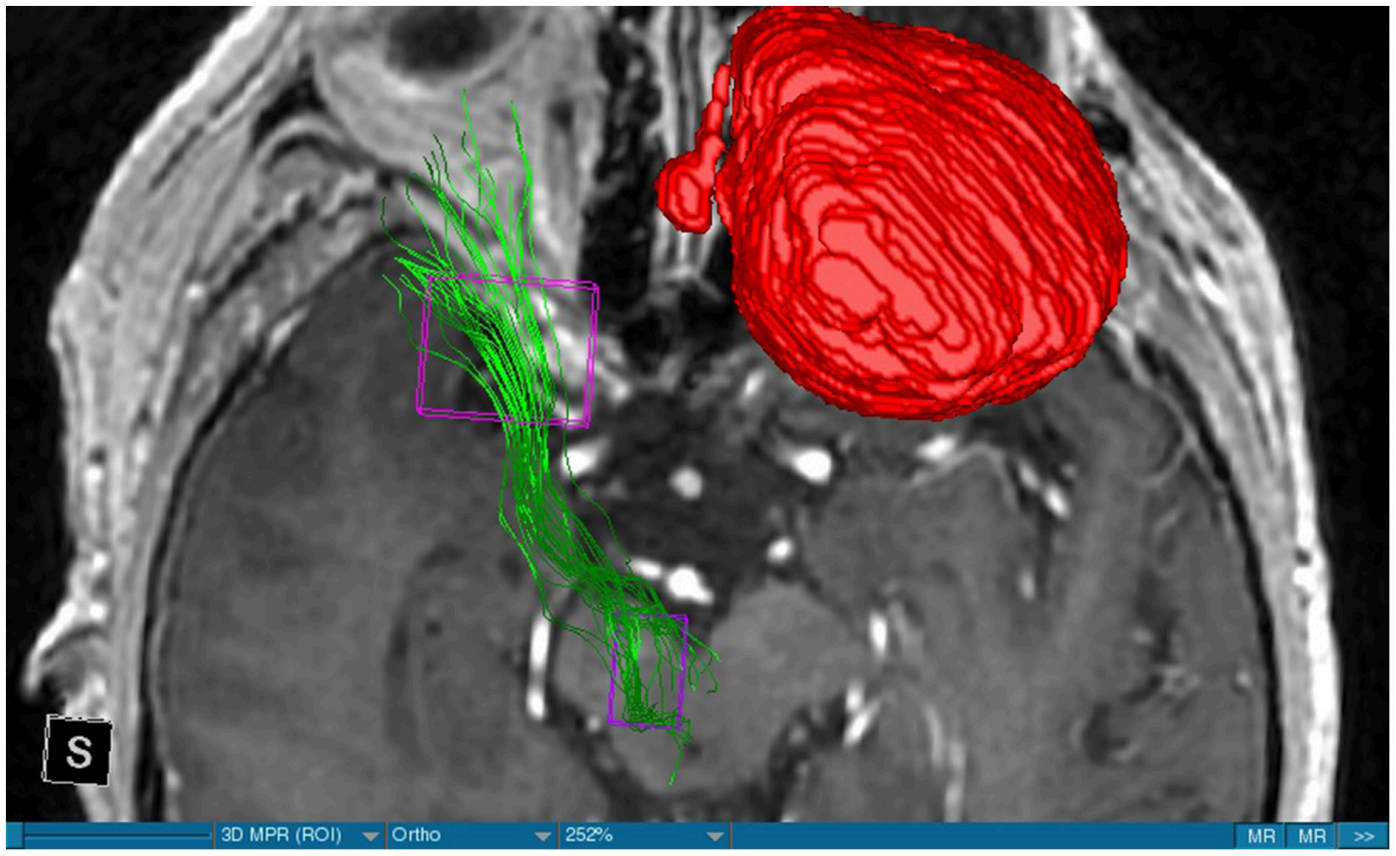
MRI axial view, T1, red: Meningioma, green: left MFB, right MFB is missing.
